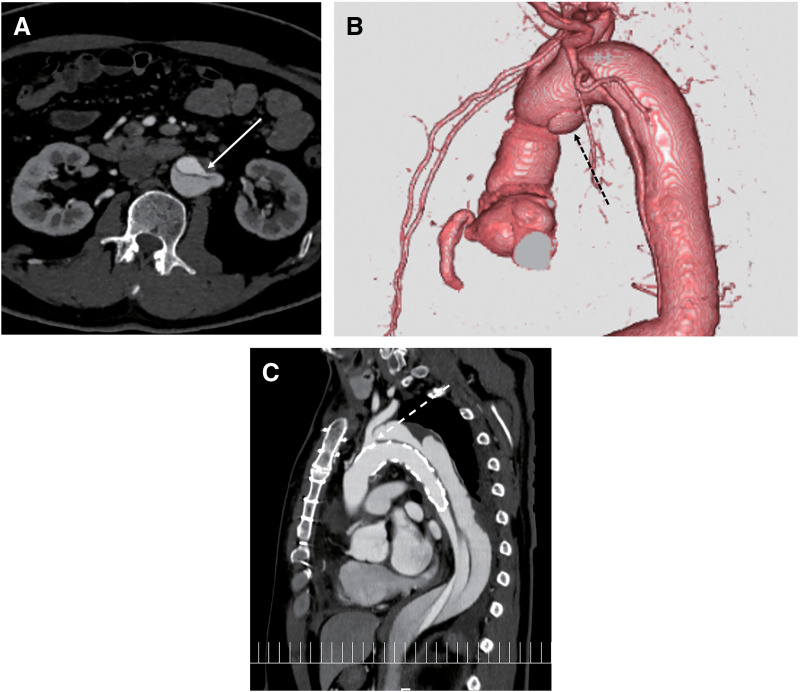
(A) Postoperative computed tomography image showing a persistent collapsed true lumen due to reentry in the abdominal aorta (white arrow). (B) Postoperative computed tomography image showing distal anastomotic new entry (black dotted arrow). (C) Postoperative computed tomography image showing a persistent collapsed true lumen due to an unresolved primary entry in the left subclavian artery (white dotted arrow) in a patient who underwent fenestrated aortic repair.
